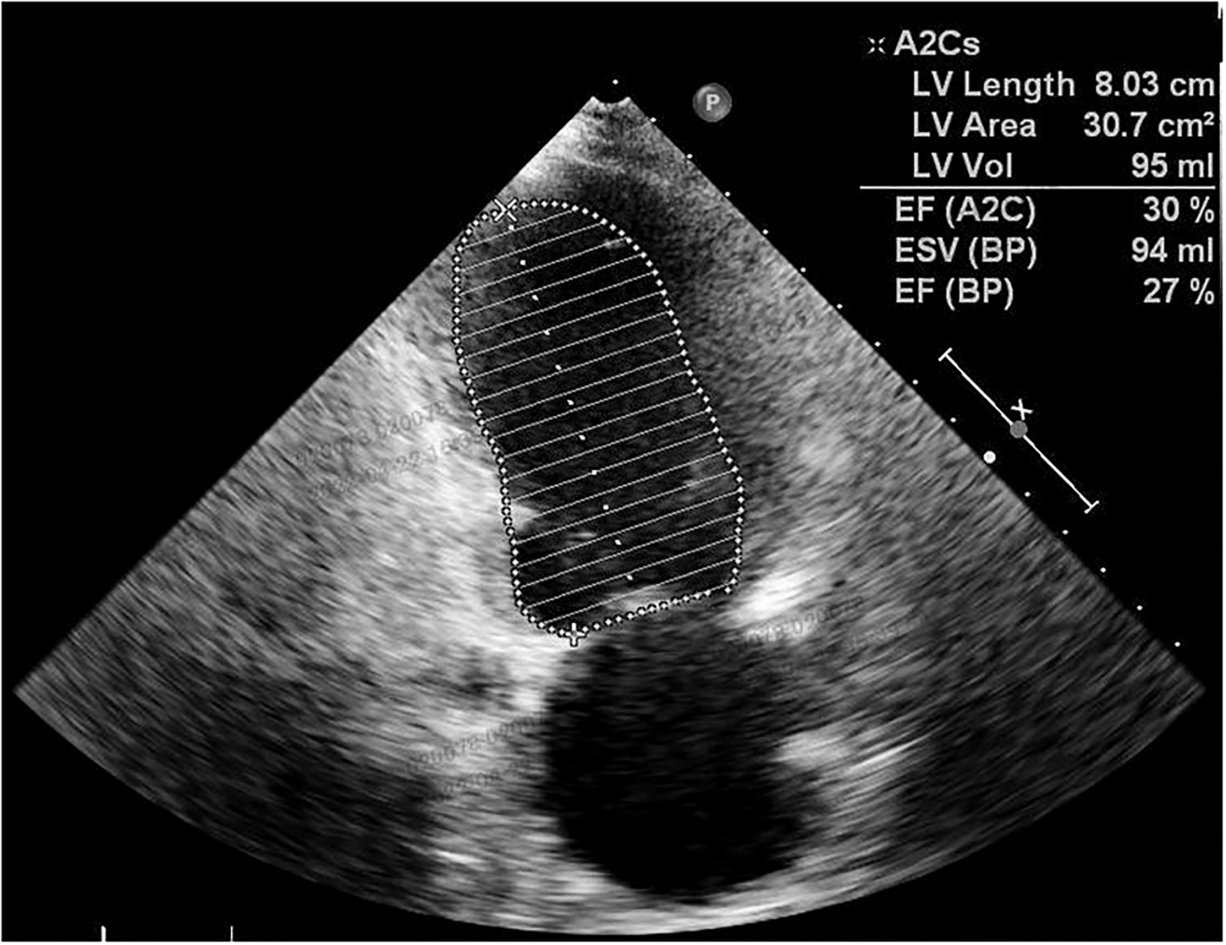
Echocardiography performed on a postoperative Day 4 showed a remarkable decrease in LVEF.
